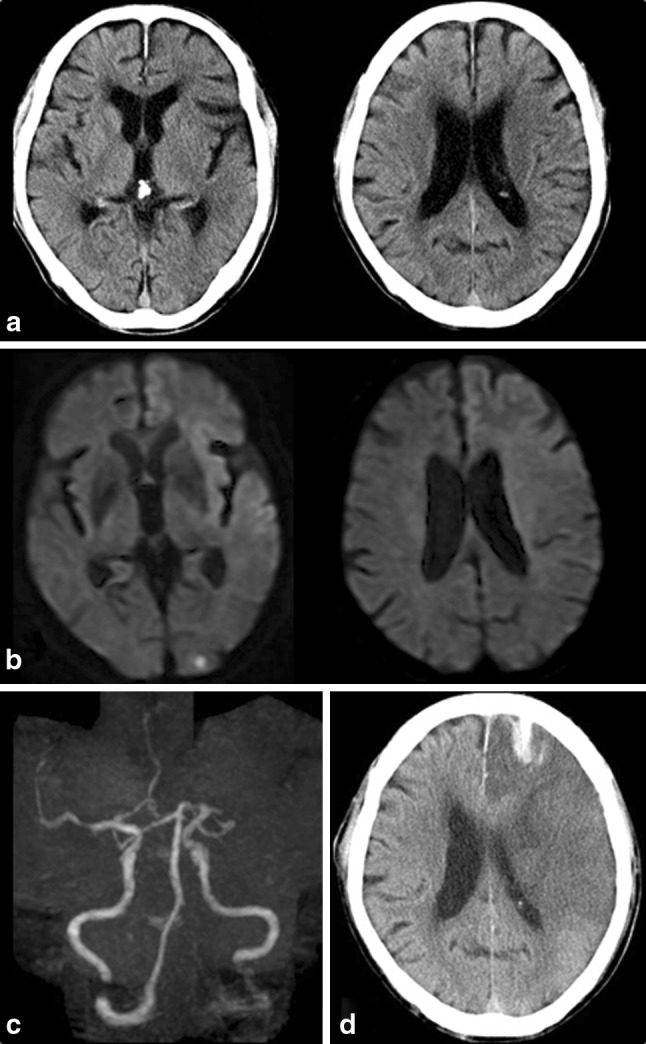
Case 2: 67-year-old man. Initial DWI at 92 min of onset shows the hyperintense lesion in the left I, M1, M2, M4, M5, and W (DWI-ASPECTS, five and ASPECTS+W, five) (a). Initial CT at 108 min of onset shows early ischemic change in the left L, I, M1, M2, and M3 (CT-ASPECTS, five) (b). MRA on admission (c) shows occlusion of the left M1 proximal portion. The NIHSS score on admission was 25. The patient was not treated with tPA, but had symptomatic ICH (PH1) 18 h of onset (d). The NIHSS score after 24 h was 38. The patient died on day four
